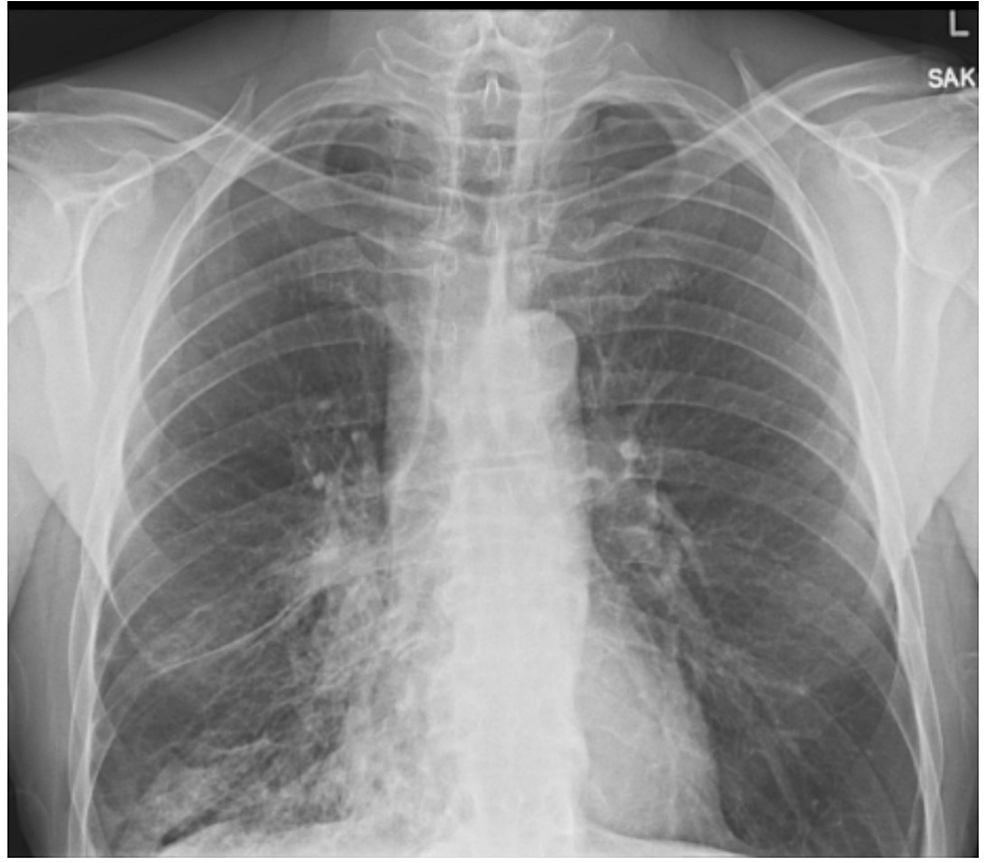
Right lower lobe pneumonia on X-ray
